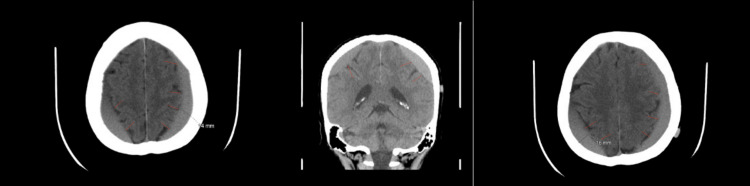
Brain CT pre-burr hole evacuation. There is an interval increase in the size and density of bilateral subdural hemorrhages, with minimal associated mass effect. The left-sided subdural hemorrhage shows a maximum thickness of 1.4 cm, compared to the previous measurement of 0.9 cm. The right-sided subdural hematoma shows a maximum thickness of 1.6 cm, compared to the previous measurement of 0.7 cm.
